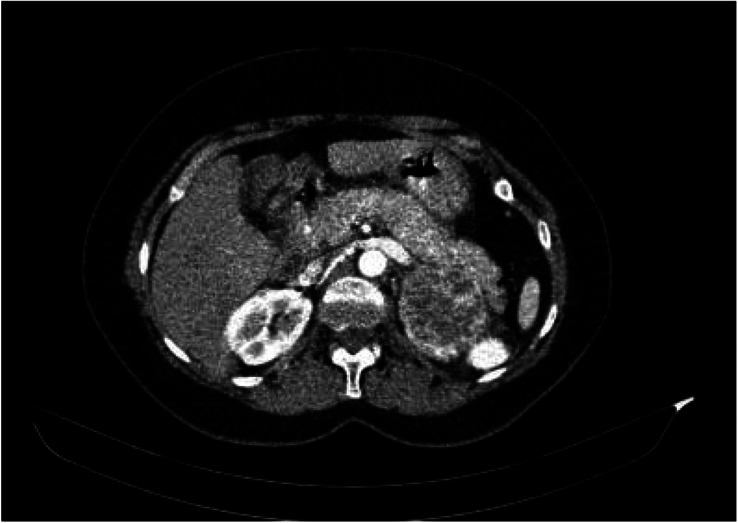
Abdominopelvic CT scan showed large, heterogeneous, enhanced, well-defined solid mass structure with a central necrotic component measuring about 64×52×68, seen on the left side of the adrenal gland.
